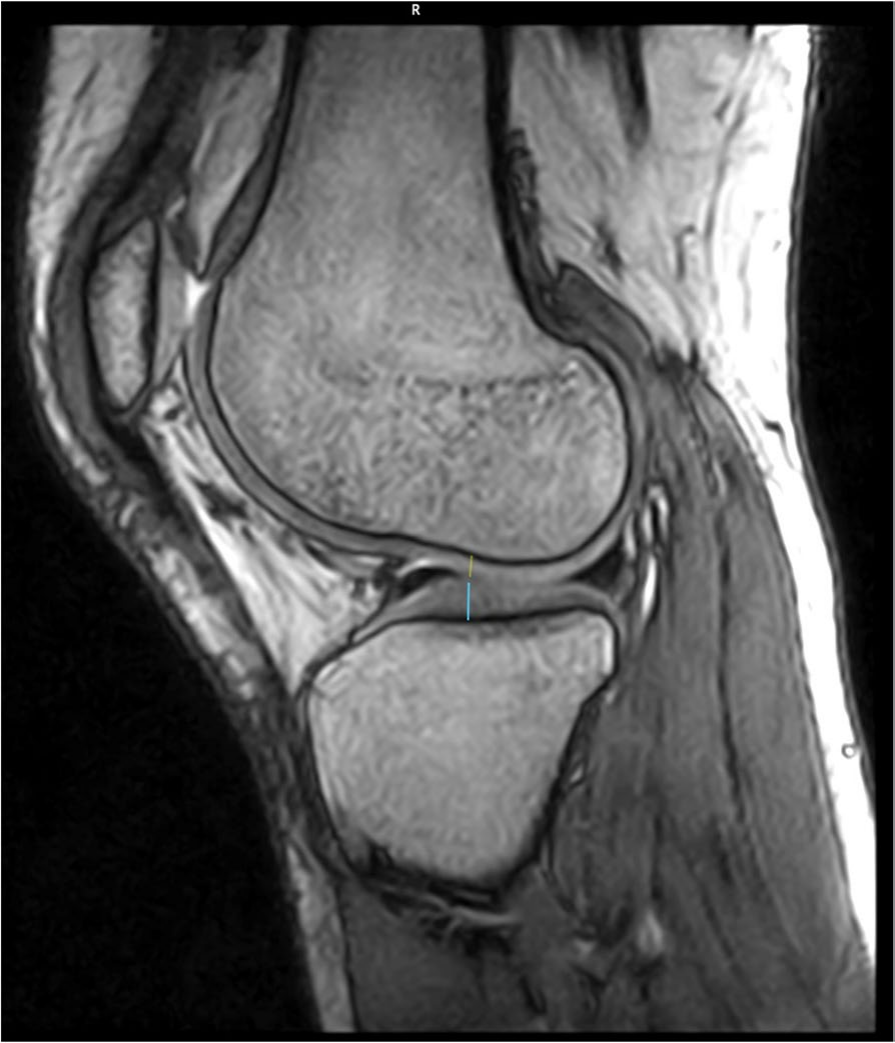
MRI cross-section with reference point for the cartilage measurement of the medial and lateral femoral and tibial condyles (saggital plane)
